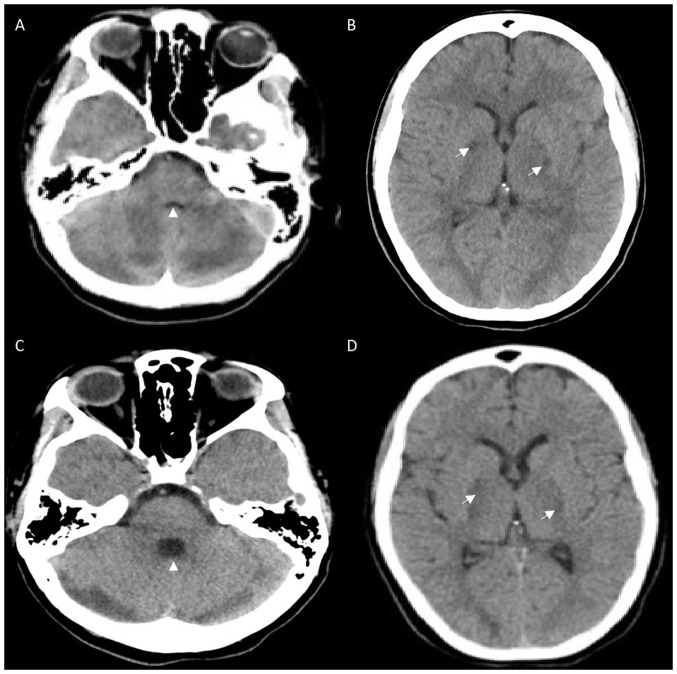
Computed tomography: (A and B) Day 1, and (C and D) day 13. (A) Loss of gray and white matter differentiation in the cerebellar hemispheres, with mass effect on the fourth ventricle (white arrowhead) are observed. (B) Bilateral hypodensities in the globus pallidus (white arrows) are observed. (C) The resolution of gray and white matter changes in the cerebellar hemispheres can be seen. No mass effect on fourth ventricle (white arrowhead) can be observed. (D) Decreased attenuation of bilateral hypodensities in the globus pallidus can be seen (white arrows).
